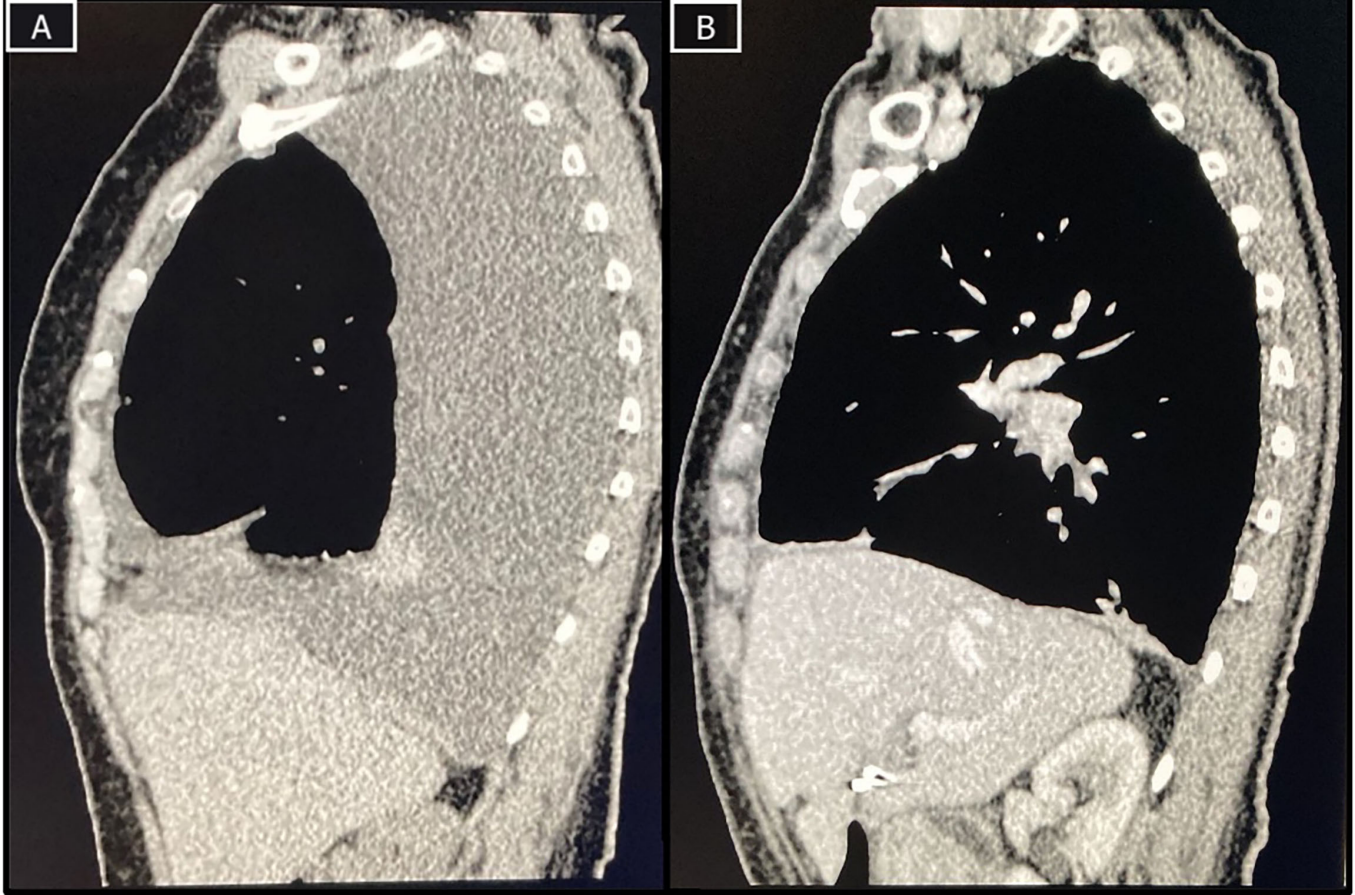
CT-scans at baseline and after surgery. (A) Baseline CT-scan demonstrating pleural effusion, thickening and nodules and a rounded atelectasis; (B) Restaging CT-scan with improvement of pleural effusion and minimal focal pleural thickening.
